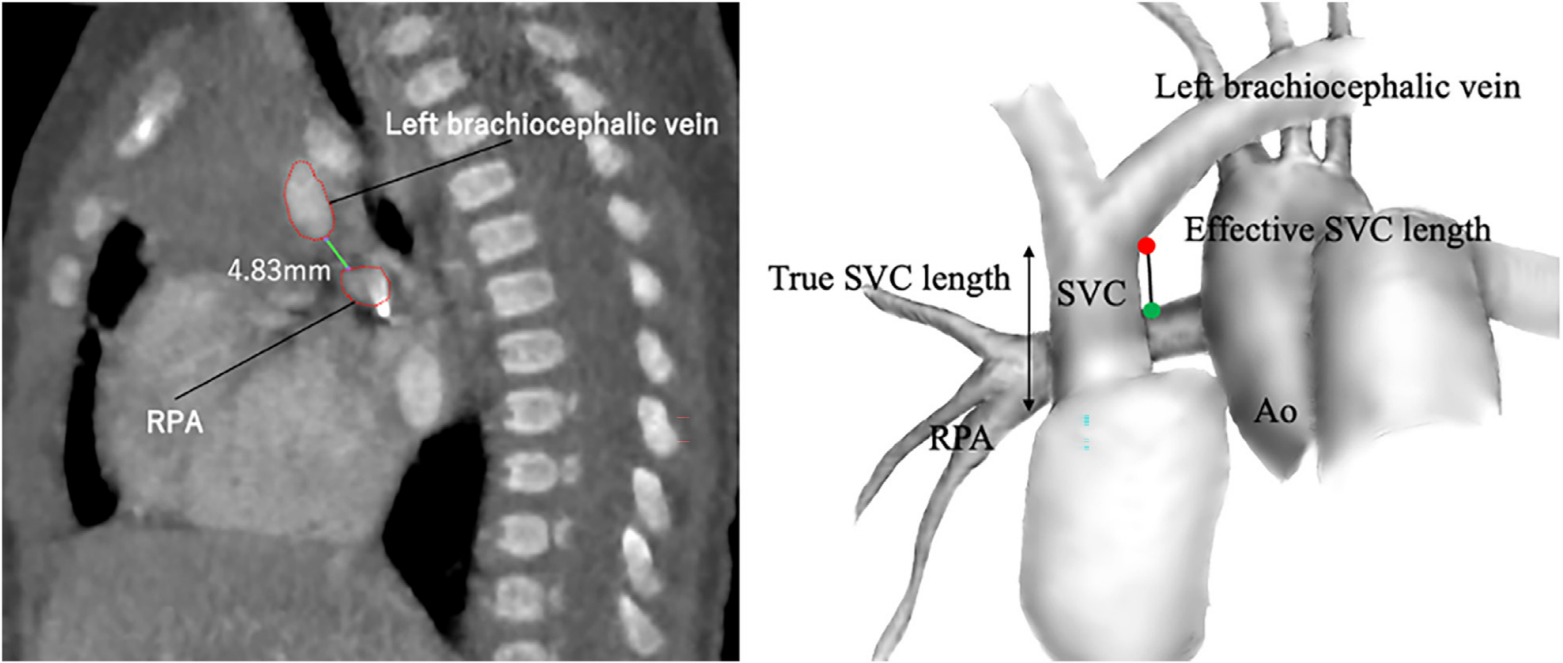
Sagittal computed tomography (CT) image of a patient with hypoplastic left heart syndrome and its variant. Green line in the CT image indicates the effective superior vena cava (SVC) length, which is defined as the distance between the red and green points in the image. (Ao, ascending aorta; RPA, right pulmonary artery.)
